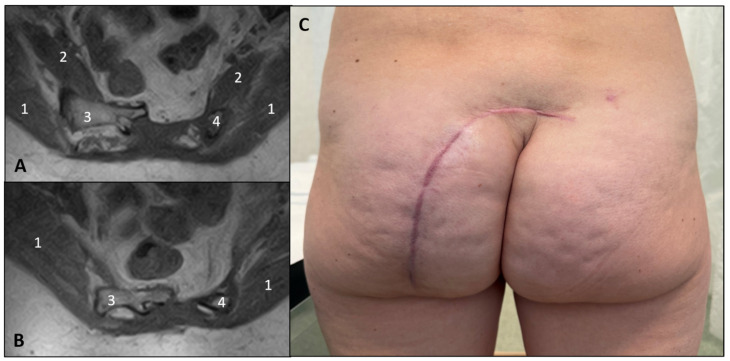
(A,B) Post-operative Magnetic Resonance Imaging (MRI) at six months. Axial T1-weighted MRI cuts ((A): cranial, (B): caudal) confirm complete lesion excision with no evidence of residual or recurrent tumor. The images effectively demonstrate the successful preservation of adjacent soft tissues and the biomechanical structures. Specifically highlighted are: (1) the symmetrical and preserved bulk of the gluteus maximus muscles; (2) the intact piriformis muscles; (3) the sacral body and right side of the sacrum; and (4) the preserved left lateral sacral margin (the ‘frame’ branch). (C) The patient’s posterior low back and gluteal region at the six-month follow-up show the well-healed arciform incision line and the symmetrical shape and trophism of the gluteal muscles, indicating successful preservation of functional anatomy and optimal cosmetic outcome.
